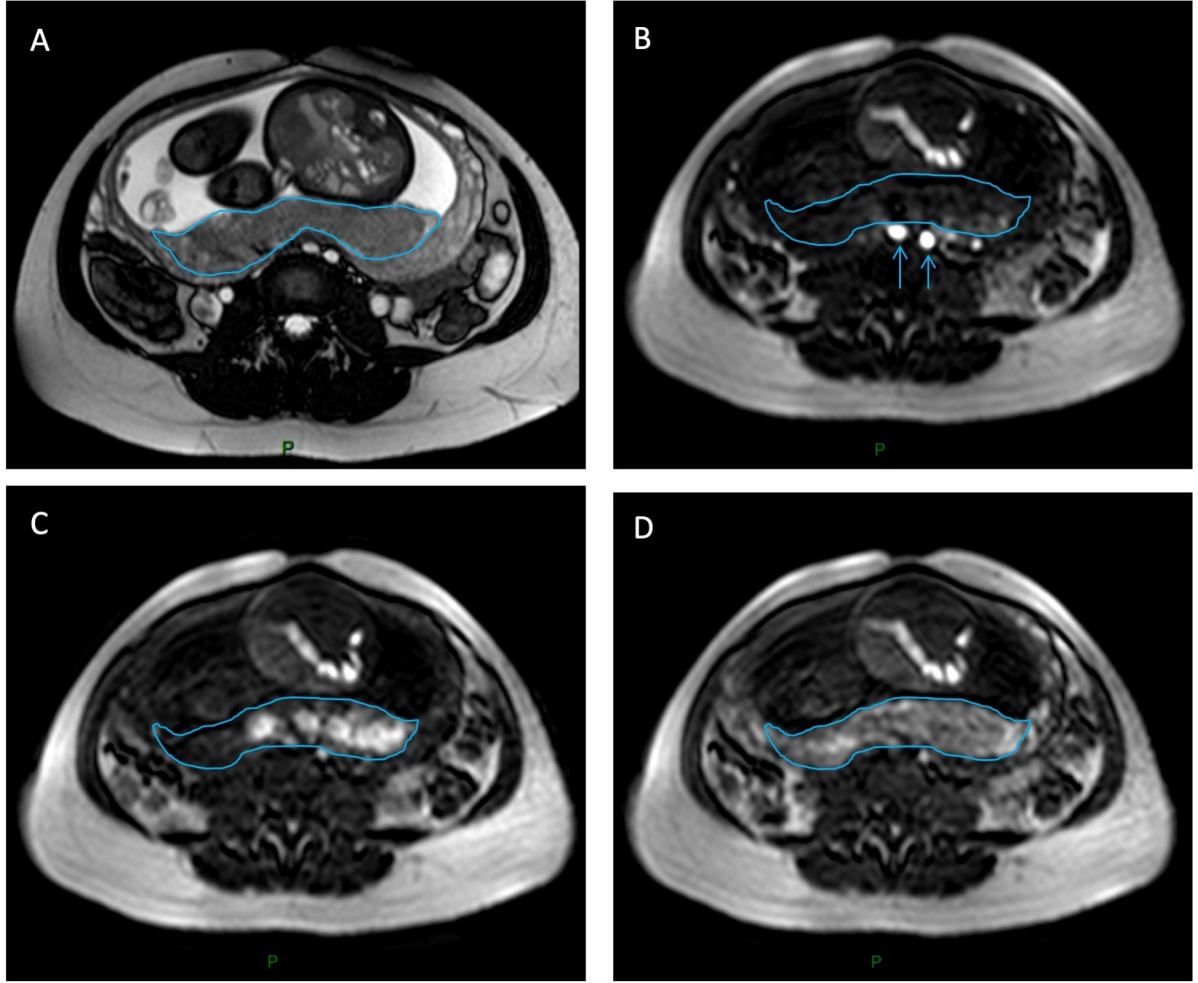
DCE MRI in the axial plane. A, shows a morphologic SSFP/FIESTA/TRU-FISP sequence. B, FSPGR image showing the enhancement in the iliac arteries after an intravenous bolus Gadolinium-chelate (blue arrows) and C, D, the enhancement of the placenta appears as high signal on these T1 FSPGR images (blue outline). After compartmental analysis of the enhancement, functional DCE MRI parameters could be evaluated.
